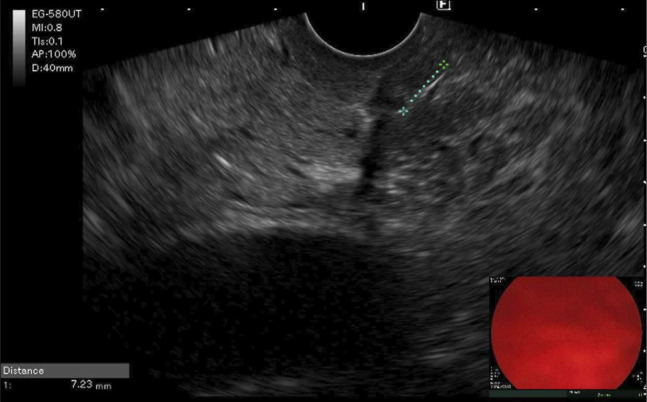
Endoscopic ultrasound image showing a sharp hyperechoic linear object traversing the bile duct.
